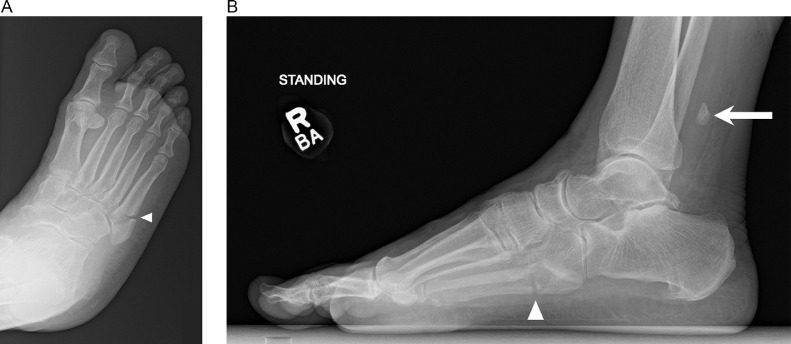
(A) Oblique and (B) lateral radiographs of the right foot 8 months after initial presentation demonstrate a fifth metatarsal base fracture (arrowhead). The os peroneum remains abnormally positioned at level of the distal fibula (arrow).
